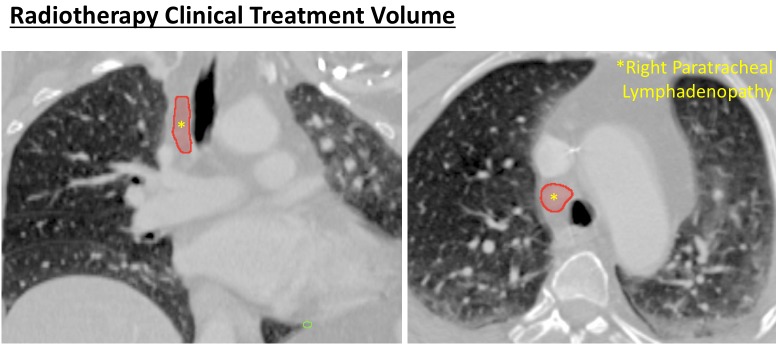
Pre-radiotherapy CT scan showing right paratracheal clinical treatment volume (CTV). CTV is outlined in red and indicated with yellow asterisk.
